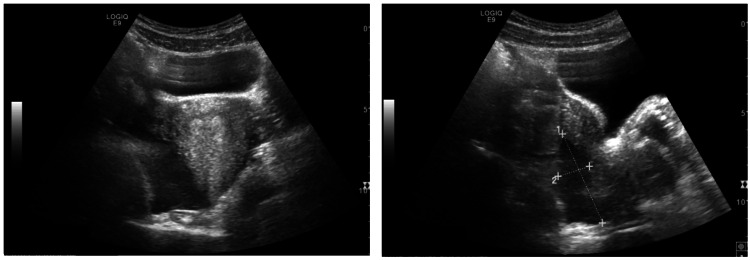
Pelvic ultrasound showed a normal-sized uterus with endometrial thickening (22 mm), normal ovaries, and a moderate amount of complex free fluid in the pouch of Douglas, measuring 55 × 18 mm in the mid-sagittal plane.
